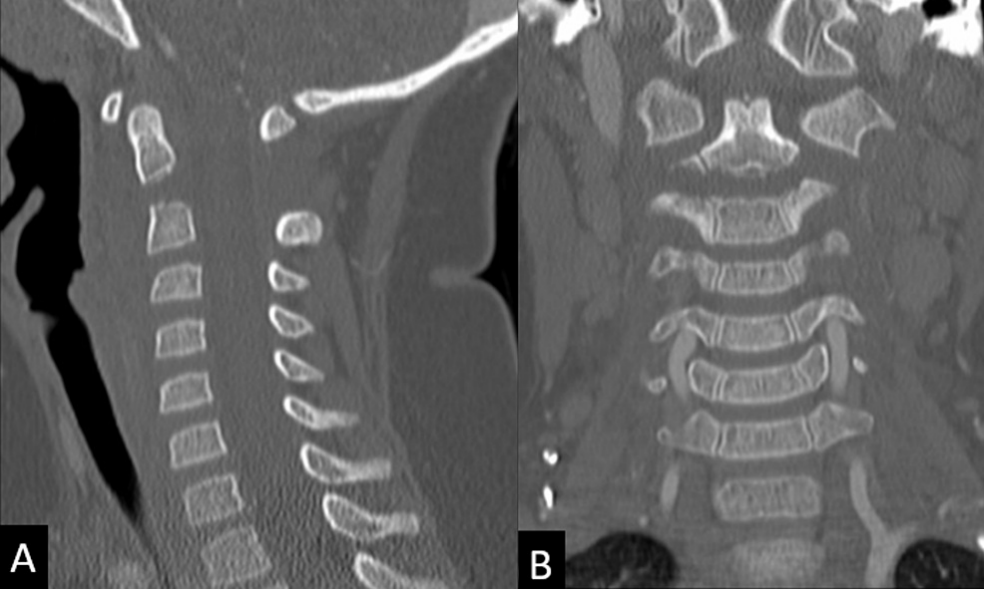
Sagittal (A) and coronal (B) CT cervical spine images demonstrating a fracture through the central odontoid synchondrosis with posterior angulation and 5mm of distraction.
